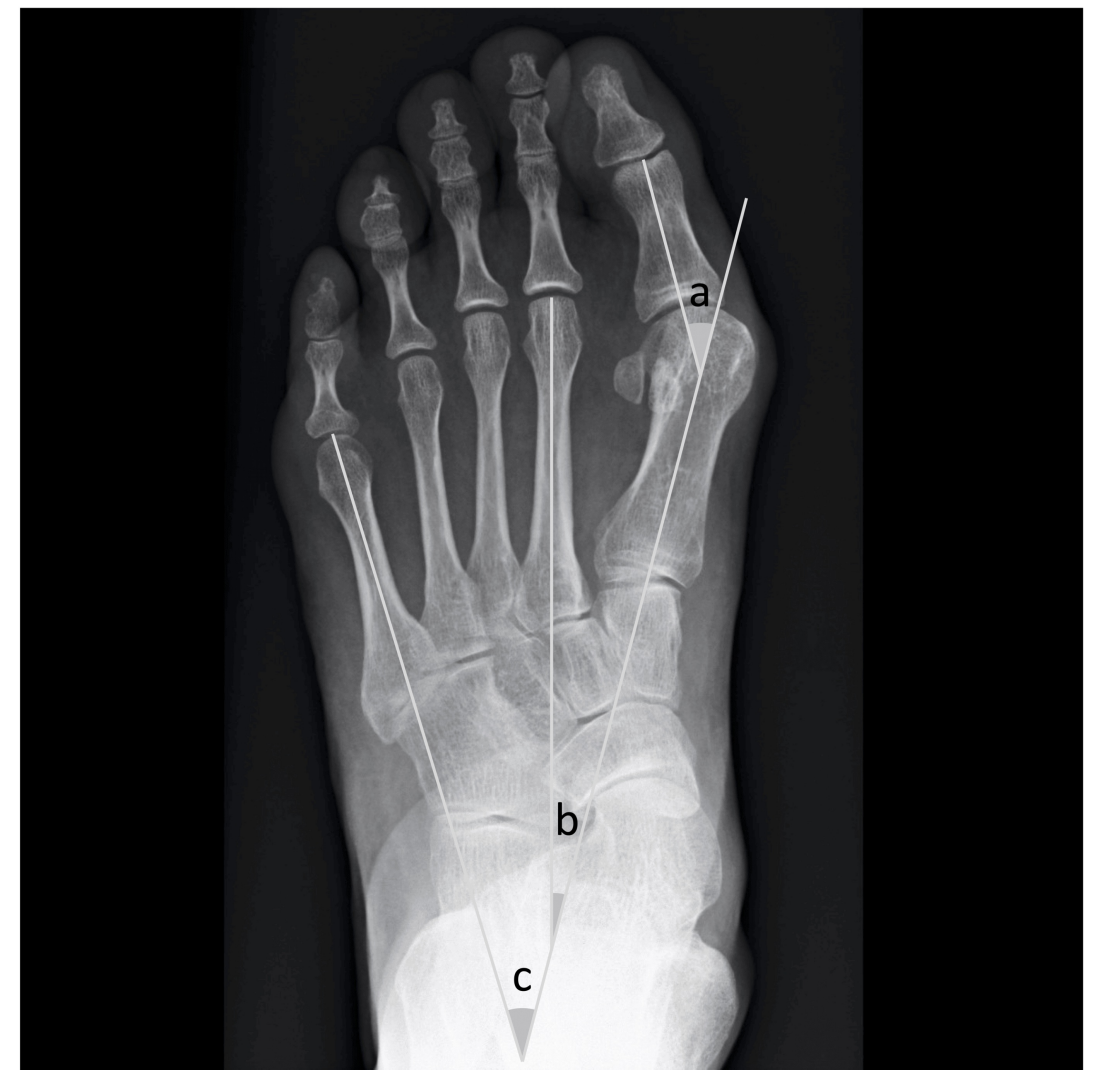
The radiographic parameters of HV, including the HVA, the M1-M2 angle, and the M1-M5 angle. The HVA refers to the angle, which is formed by the axis of the proximal phalanx of the hallux and the axis of the first metatarsal (a). ﻿The M1-M2 angle is the angle, which is formed by the longitudinal axis of the first and second metatarsals (b). The M1-M5 angle is the angle, which is formed by the longitudinal axis of the first and fifth metatarsals (c). HV: hallux valgus, HVA: HV angle.
